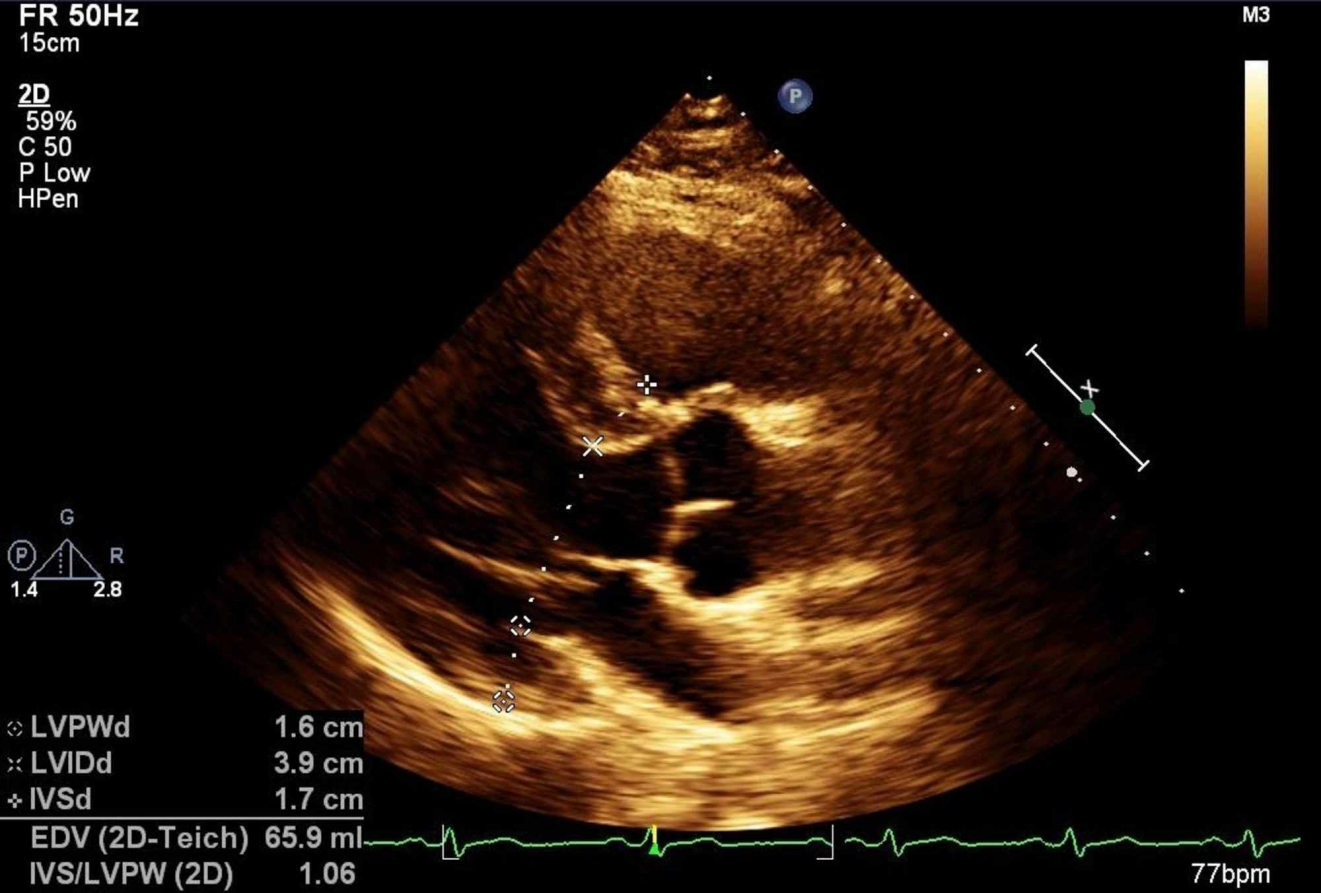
TTE demonstrating moderate LVH with septal wall thickness of 18 mm and posterior wall thickness 17 mm. Chordal SAM is observed. TTE, transthoracic echocardiogram; LVH, left ventricular hypertrophy; SAM, systolic anterior motion.
